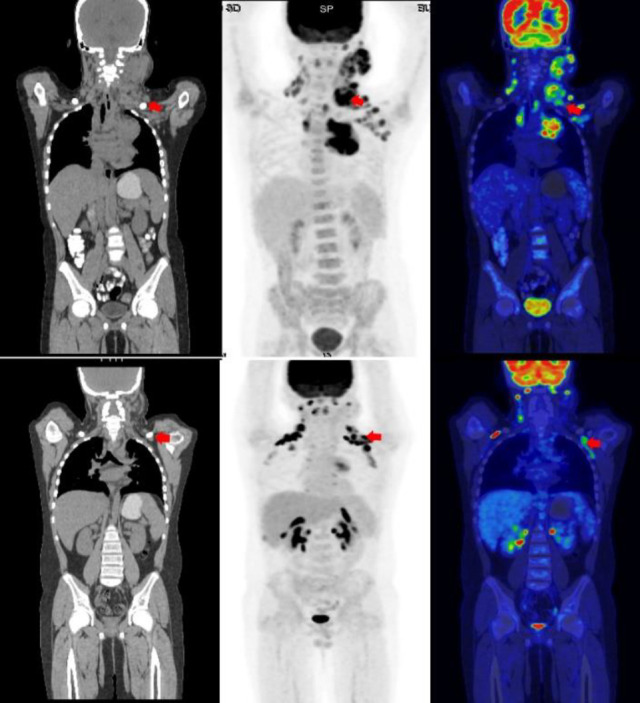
A 13-year-old male patient was diagnosed with classical Hodgkin lymphoma (cHL), mixed cellularity type, stage IIIA. Baseline PET/CT demonstrated FDG-avid lymphadenopathy in cervical, mediastinal, and axillary regions (SUV_max_: 23.4) (A-C, red arrow). After receiving 2 cycles of ABVD chemotherapy, interim PET/CT (iPET) showed significant reduction in lesion size but with residual metabolic activity exceeding liver uptake (Deauville score 4, partial metabolic response [PMR]), consistent with iPET-positivity (D-F, red arrow). Treatment was subsequently intensified to 4 cycles of BEACOPP, achieving complete metabolic response (CMR). The patient has maintained sustained remission without evidence of disease recurrence during follow-up
